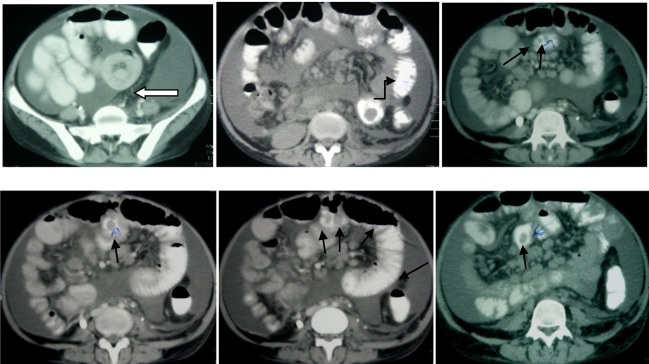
The patient's abdominopelvic CT scan showing the mass causing a non-obstructing intussusceptions (hollow arrow) and pedunculated polypoid lesions of the small intestine (black arrows).
